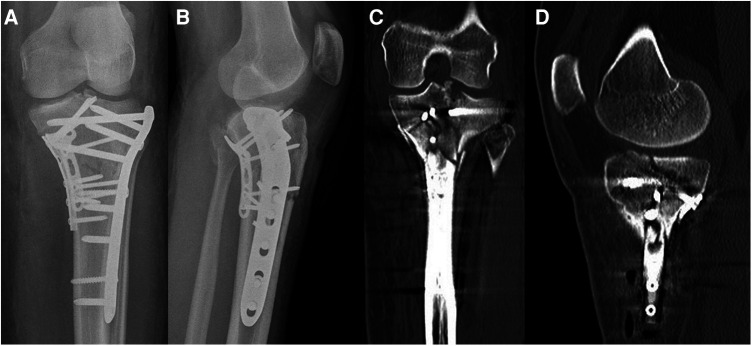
Case 5, 3-column fracture after revision surgery (posteromedial extension of the pre-existing medial approach, direct reduction and fixation of the split wedge fragment with a 3.5 mm T-plate), AP (A) and lateral (B) x-ray, coronal (C) and sagittal (D) CT reconstruction.
